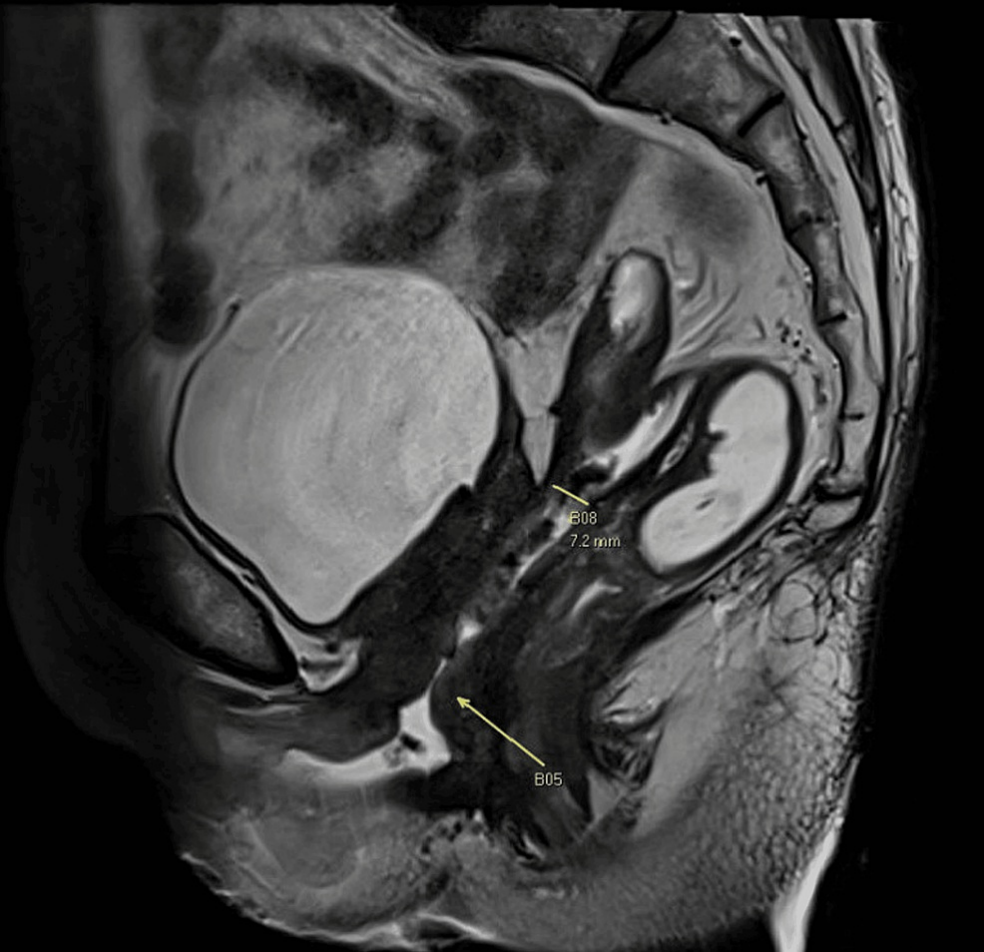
MRI pelvis showing the colovaginal fistula
